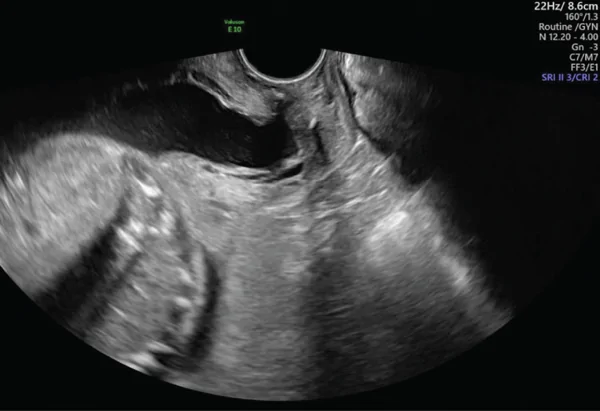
Follow‐up transvaginal ultrasound at 23 + 3 weeks demonstrating the cerclage in place, with membranes positioned above the level of the suture.
